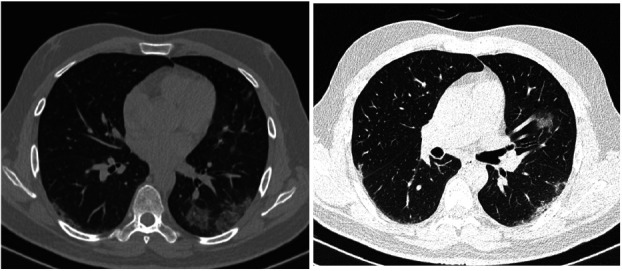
39-year-old male with history of vomiting and abdominal pain. No cough or fever. CT scan of the abdomen found incidental ground glass opacities at the lung bases for which a subsequent dedicated chest CT scan was requested showing bilateral and subpleural ground-glass opacities (Negative initial PCR testing. Positive repeat PCR within 3 days)
